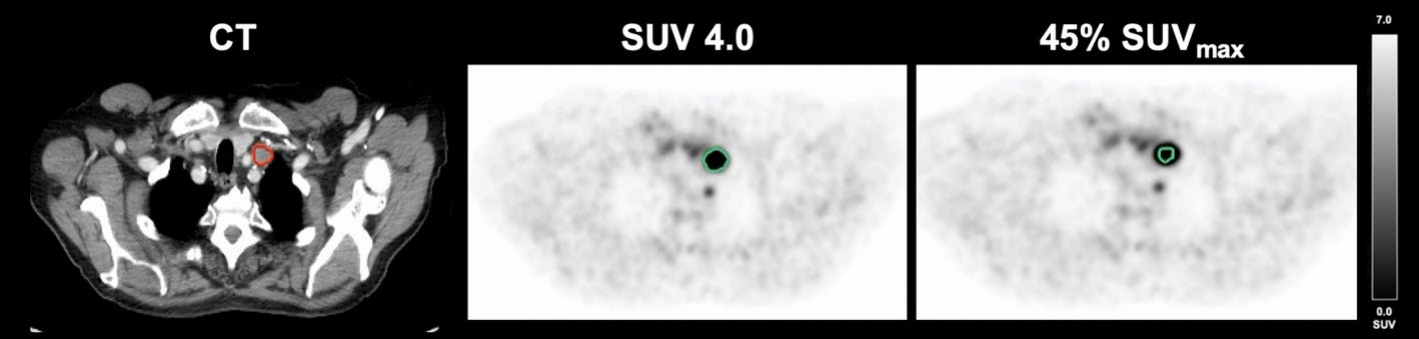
Delineation methods in a lymph node metastasis of a patient with GEPNET. Volumetric reference standard 2.91 mL; SUV 4.0: 9.19 mL; 45% SUV_max_: 2.79 mL
